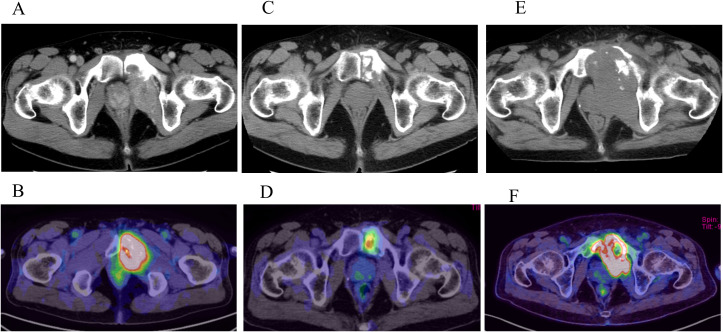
Imaging-based assessment of treatment response over time: Pretreat; computed tomography (CT) (A) and positron emission tomography (PET)-CT (B) 12 months after initiation of pembrolizumab treatment; CT (C) and PET-CT (D) 18 months; CT (E) and PET-CT (F).
